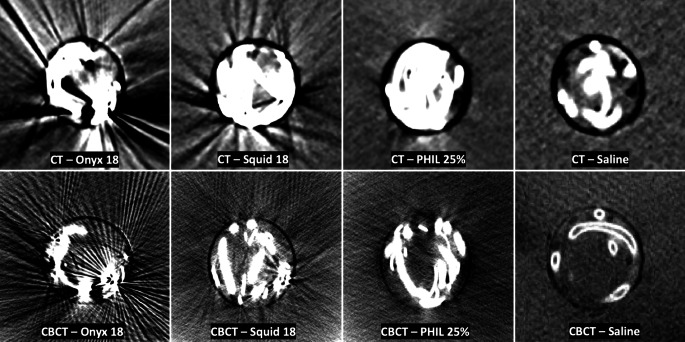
Representative CT and CBCT images of the novel AVM model in axial plane. For conventional CT a standard brain window with a width of 80 HU and a length of 40 HU and for CBCT a width of 350 DU and a length of 30 DU was set. A higher degree of imaging artifacts for the EVOH-based LEAs (Onyx and Squid) was observed compared to PHIL which uses iodine as its radiopaque component
